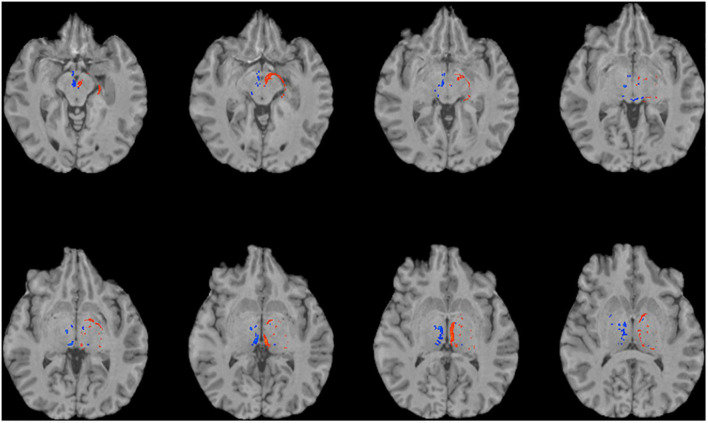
Using single-shell “clinical grade” dMRI data to reconstruct the afferent and efferent tracts of the LHb. Axial T1 MR images with superimposed streamline density maps of LHb fiber tracts are shown for a representative subject. Single-shell/lower *b*-value dMRI does not reconstruct the streamlines as faithfully as the multi-shell/higher *b*-value data. Left hemisphere streamlines are colored in red and right hemisphere streamlines are colored in blue.
